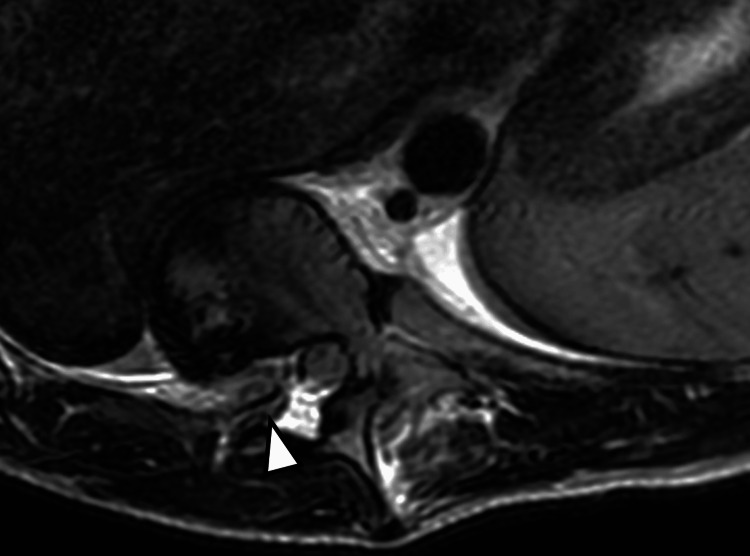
Axial T2-weighted image through T10-T11 level showing protrusion of the right T11 rib head through the neural foramen into the spinal canal. There is no cord compression or signal abnormality at this level. Note that findings of rib herniation can be subtle on MRI, and close attention and familiarity with this condition are essential to identify this finding, particularly in patients with NF1-associated dystrophic scoliosis.
